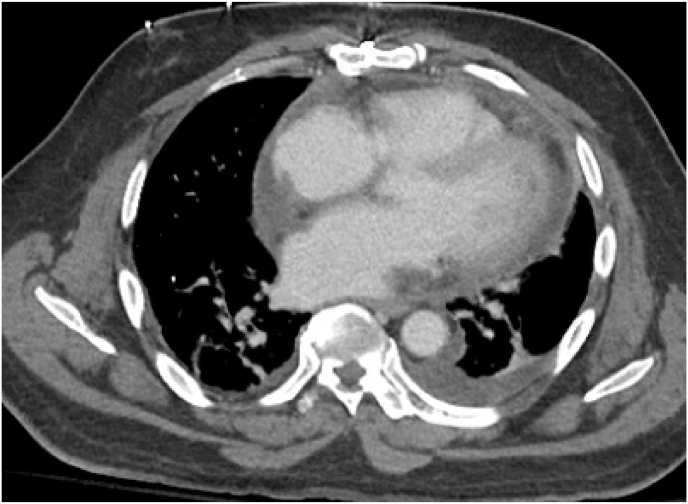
CT image of the thorax with loculated pericardial effusion.
